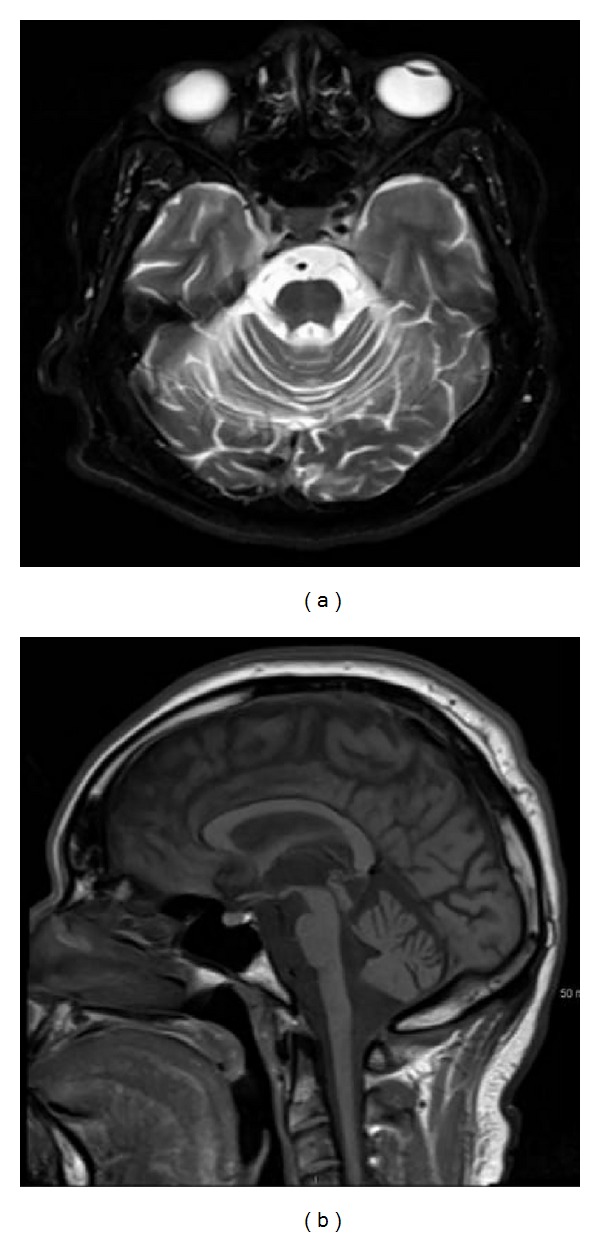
MRI of IV:10, an axial T2 on the left and sagittal FLAIR on the right, demonstrating pontine and cerebellar atrophy.
